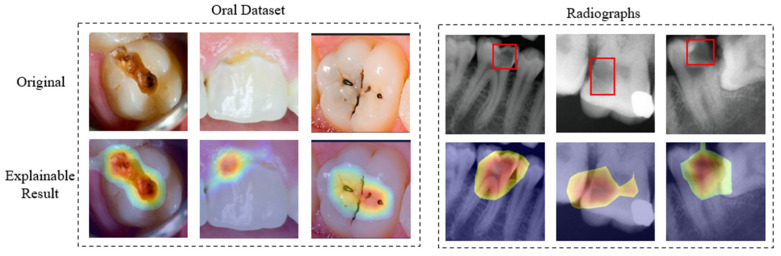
XGrad-CAM visualizations for representative samples from the (left) oral photograph dataset and (right) radiograph dataset, with the top 30% and 20% of pixels highlighted, respectively. The area within the red box indicates the approximate extent of the dental caries.
